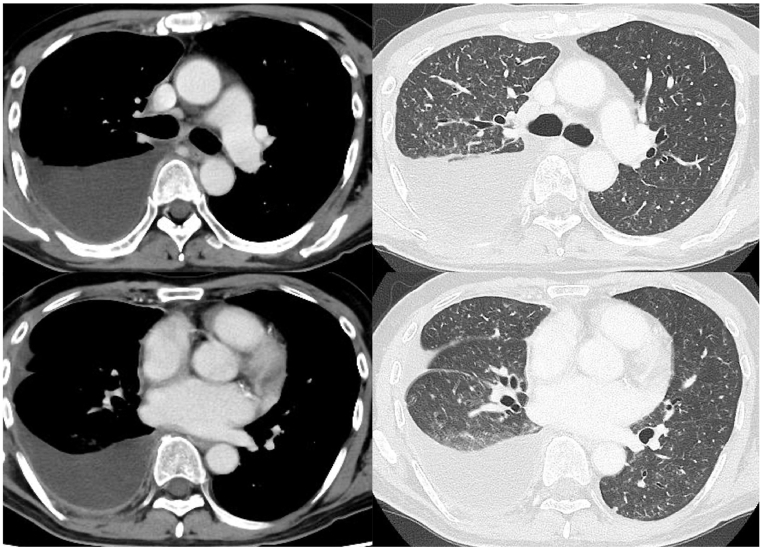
Chest computed tomography scan revealed right pleural effusion and multiple micronodules with a random distribution in both lung fields.
